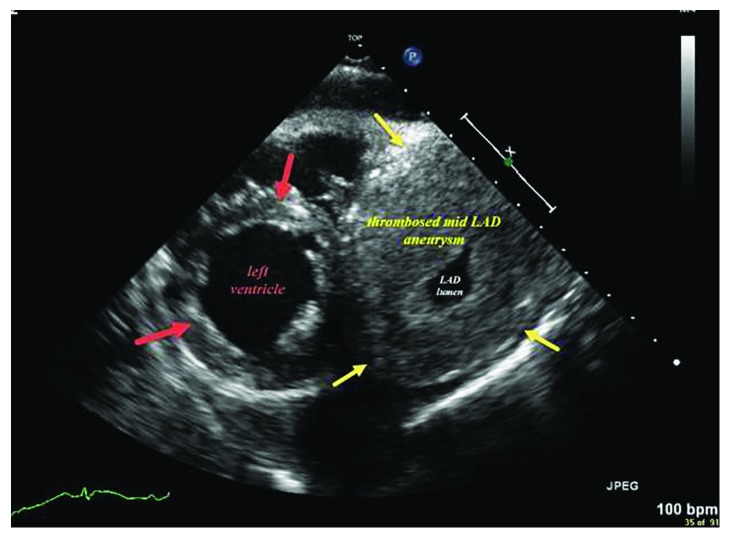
Two-dimensional transthoracic echocardiogram short axis view showing a large extracardiac mass (yellow arrows) alongside the left ventricle (red arrows).
